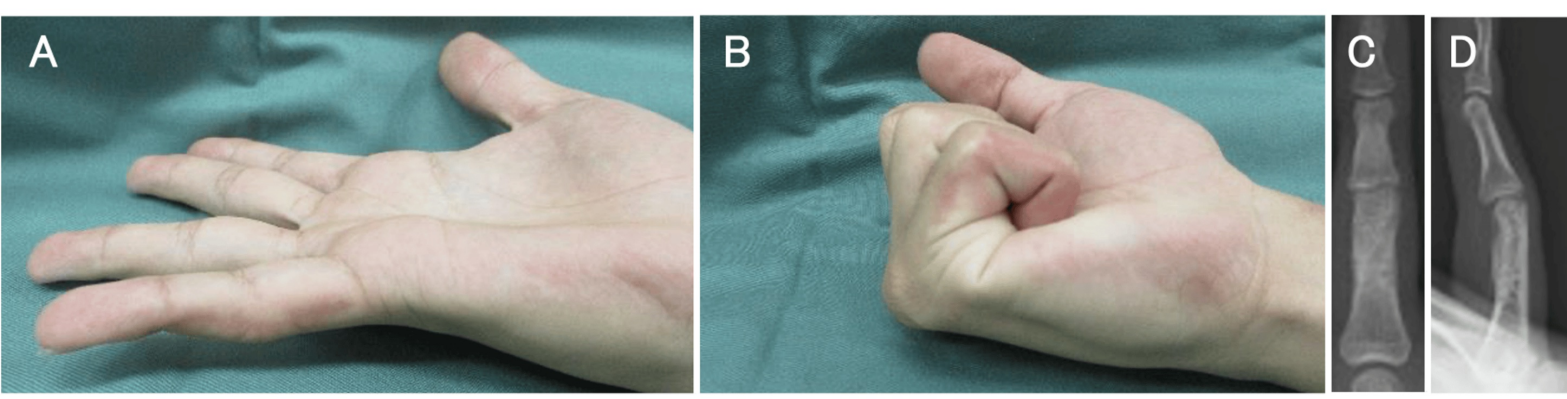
Photographs at the nine-month follow-up and radiographs at the 12-month follow-up. (A, B) Photographs showing good active range of motion of the finger at the nine-month follow-up. (C, D) Radiographs following implant removal 12 months after osteotomy, showing bone union at the proximal phalangeal fragment with good bony alignment.
